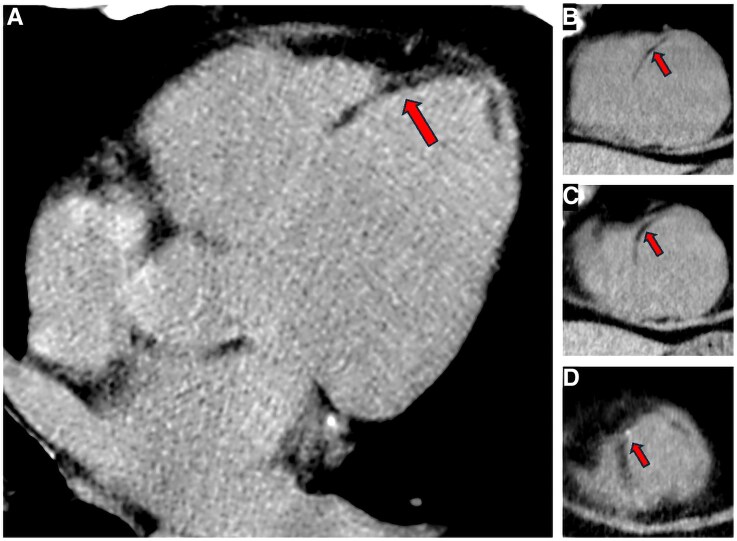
Lipomatous metaplasia visualized using dual-energy CT. The panels show the post-contrast dual-energy CT images processed for the energy threshold of 40 keV in long axis four-chamber view (*A*) as well as short axis views of the basal (*B*), mid (*C*) and apical (*D*) segments. Red arrows indicate the regions with lipomatous metaplasia. Abbreviations: CT – Computed Tomography.
